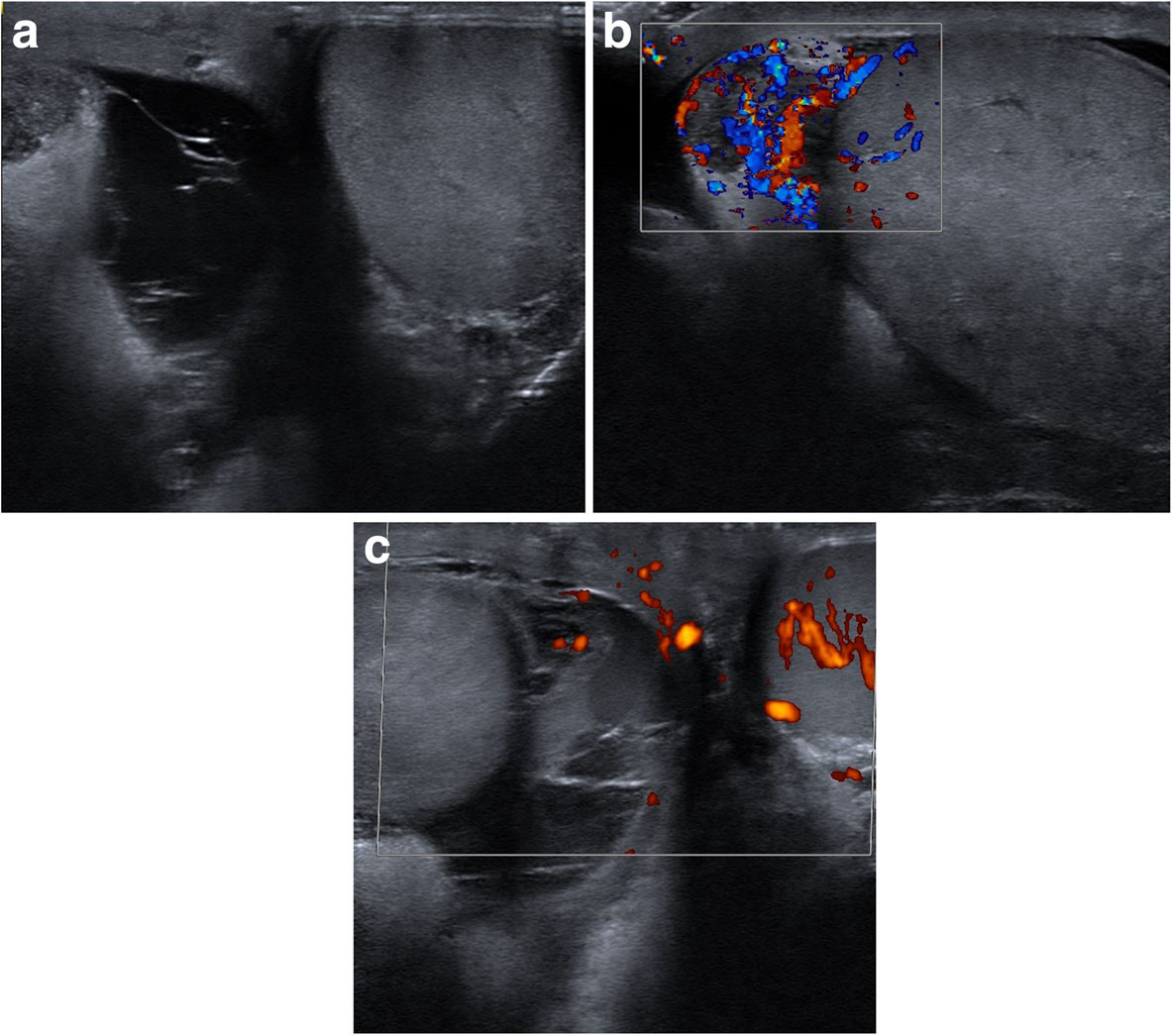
a-b: Initial ultrasonographical images with a: Pyocoele, b: accentuated doppler of epididymo-orchitis. c: Images of the second ultrasonographical evaluation, with right-sided avascular necrosis with pyocoele in comparison to a normal left testis
